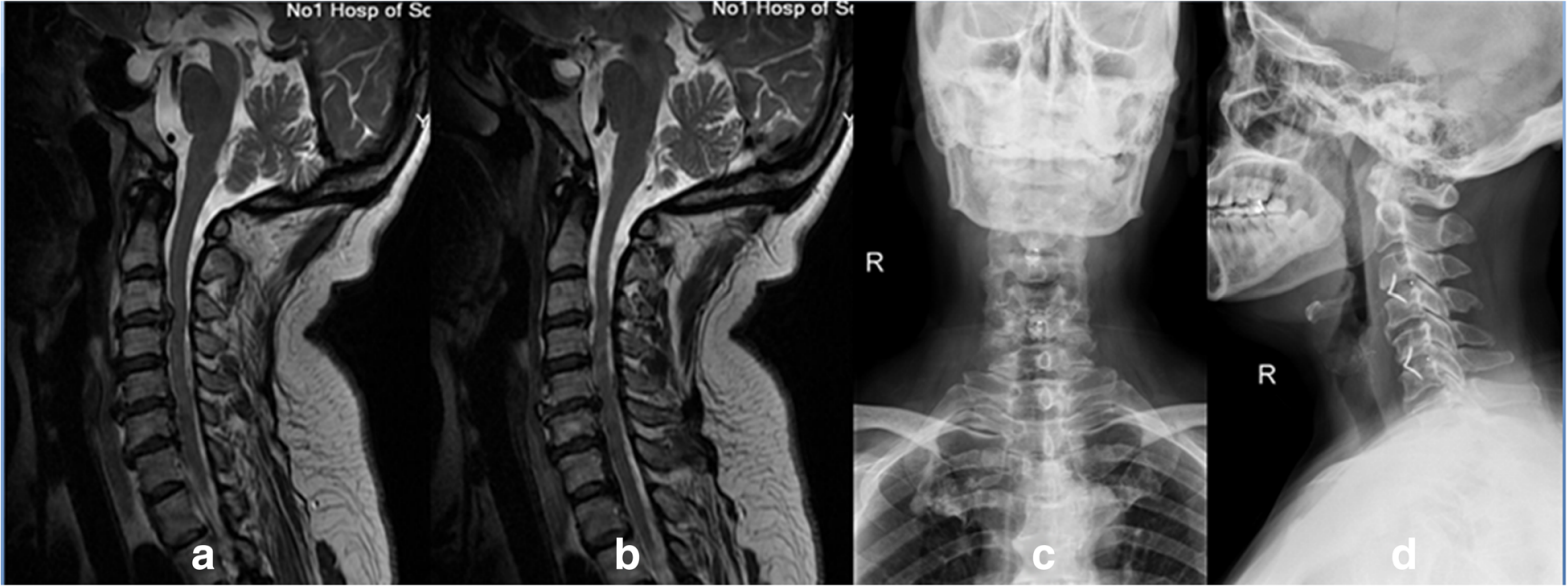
Preoperative lateral T2-weighted MRI (a and b) showing a 59-year-old woman with C3-C4 and C5-C6 disc herniation. Anteroposterior (c) and lateral (d) radiographs showing C3-C4 and C5-C6 anterior cervical discectomy and fusions (ACDF) with the zero-profile anchored spacers
